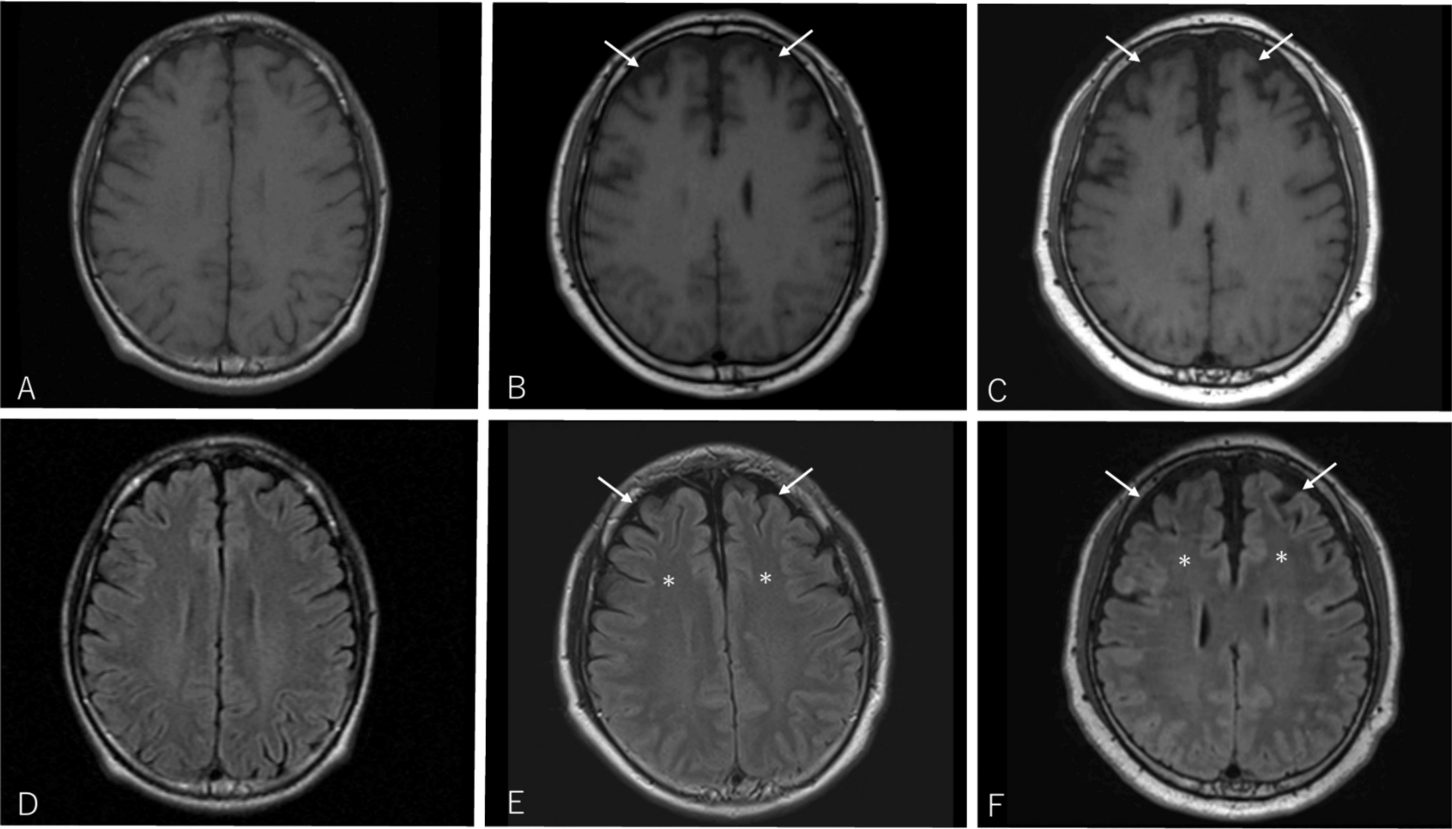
Serial Brain MRI Findings Over 9 Years Brain MRI of the patient in 2011 (A, D), 2015 (B, E), and 2020 (C, F). Serial T1-weighted (A–C) and FLAIR (D–F) images show progressive cortical atrophy in the bilateral frontal lobes over time. Arrows indicate areas of frontal cortical atrophy, and asterisks denote the absence of significant high-intensity lesions on FLAIR images. FLAIR: fluid-attenuated inversion recovery
